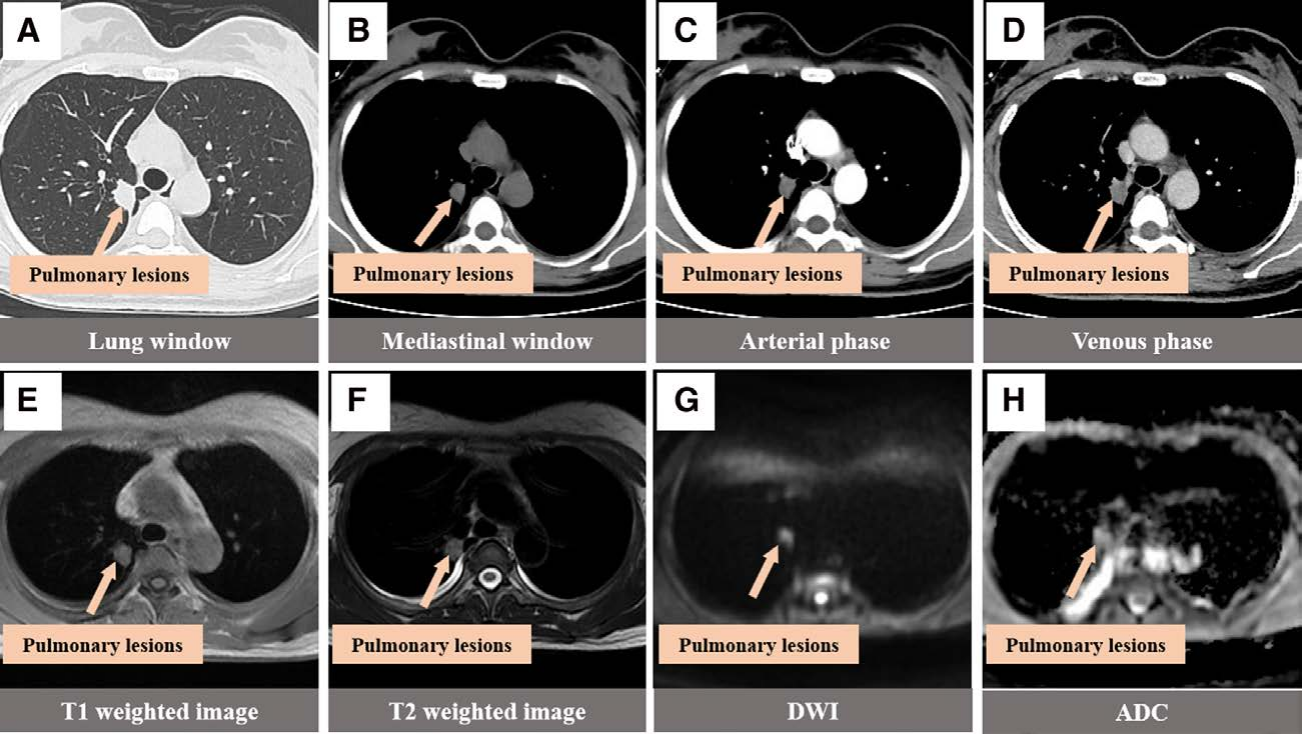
CT images (A–D) and MRI images (E–H) of the patient. (A, B) Pulmonary window and mediastinal window: oval soft tissue density nodules in the right upper lung, (C, D) enhanced arteriovenous phase: mild to moderate uneven delayed enhancement with slight thickening of adjacent pleura on enhanced scan. MRI images were injected with oval, T1 and slightly longer T2 signal nodules in the right upper lung of (E, F) with a small amount of pleural effusion, (G) nodules showed hyperintensity on DWI, and (H) ADC nodules showed hyperintensity. ADC = apparent diffusion coefficient, CT = computed tomography, DWI = diffusion-weighted imaging, MRI = magnetic resonance imaging.
